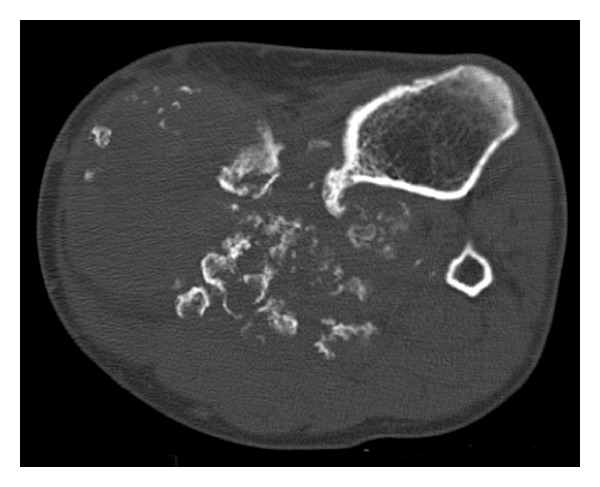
Axial CT scan of tibia and fibula demonstrating a peripheral dedifferentiated chondrosarcoma with central and peripheral, bimorphic mineralization, destruction of the prior exostosis, and a soft-tissue mass.
